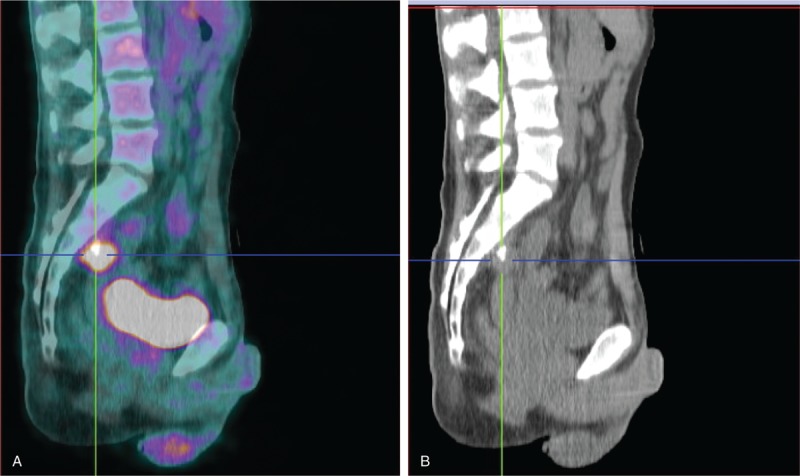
Sagittal images: fused FDG-positron emission tomography/CT (A), CT alone (B). There is increased uptake at the presacral level, suggesting local recurrence, with an SUV_max_ of 6.5. CT = computed tomography, FDG = fluorodeoxyglucose.
